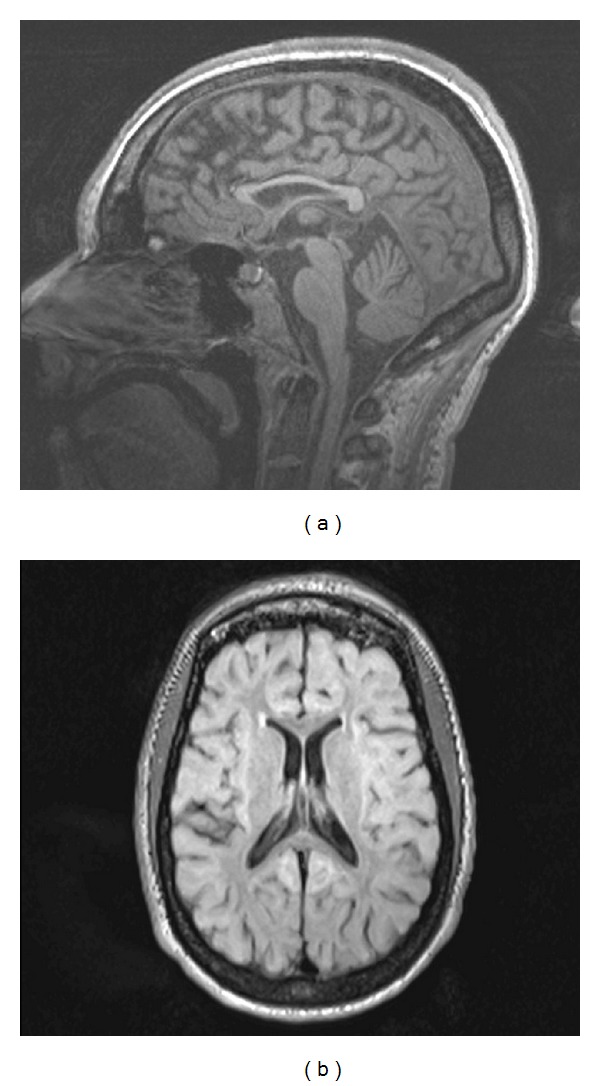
Hypoplastic corpus callosum in magnetic resonance imaging of the patient. (a) sagittal plane; (b) axial plane.
